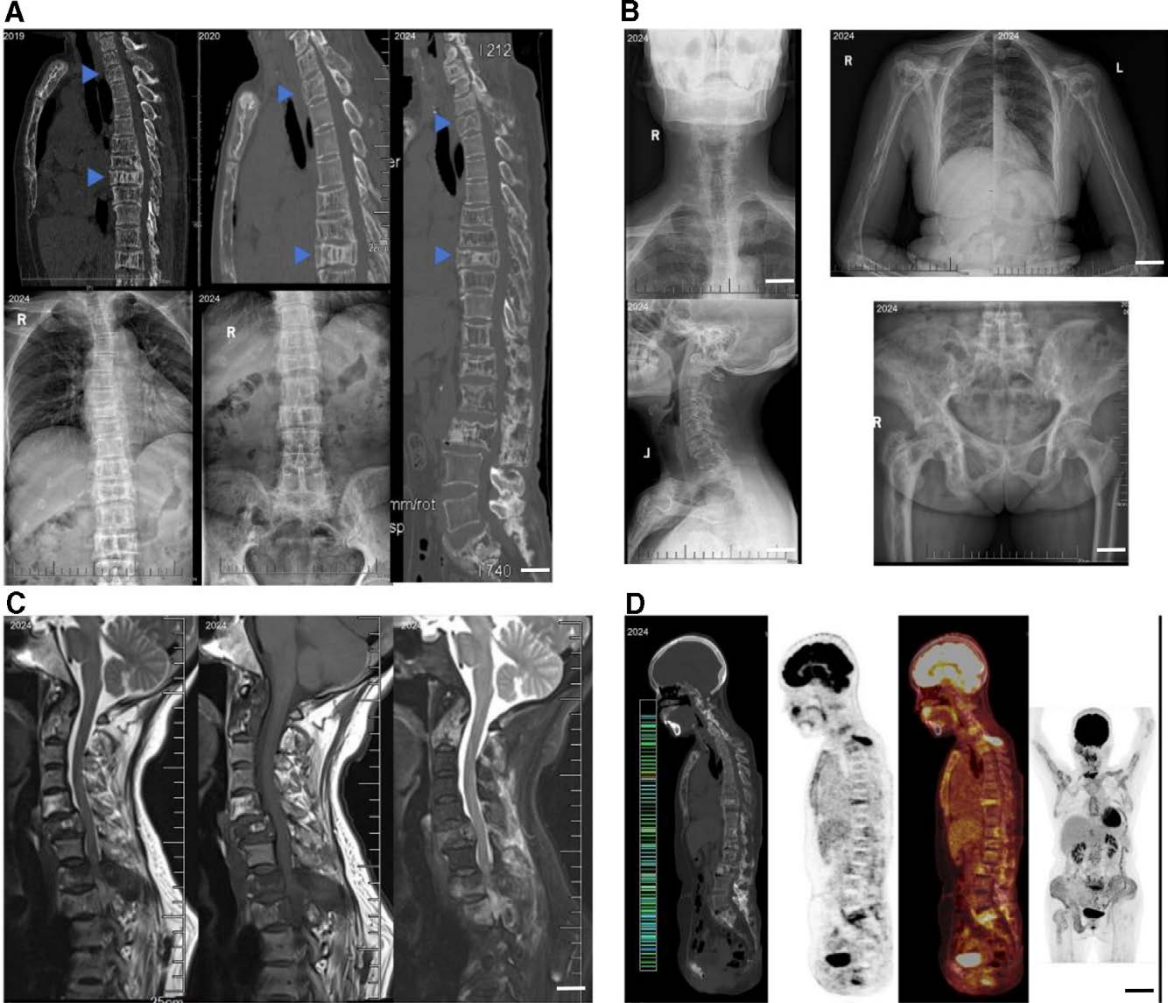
Images data across different years. (A) Thoracic CT images from different years and the most recent thoracolumbar X-ray. The blue arrows indicate vertebrae with progressive bone destruction and decreasing vertebral height (scale bar = 4 cm); (B) The most recent X-rays of the cervical spine, humerus, pelvis, and femoral neck (scale bar = 3 cm); (C) MRI of the cervical and part of the thoracic spine, showing abnormal signals in the vertebral bodies and their appendages, as well as in the surrounding soft tissues (scale bar = 2 cm); (D) PET-CT imaging of the patient’s spine (scale bar = 10 cm). CT = computed tomography, MRI = magnetic resonance imaging, PET-CT = positron emission tomography-computed tomography.
